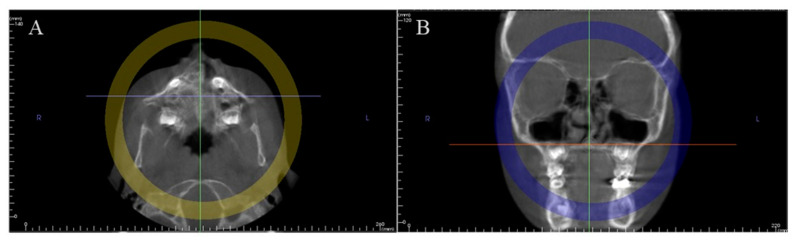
Example of a CBCT record: (A) axial view; (B) frontal view.
